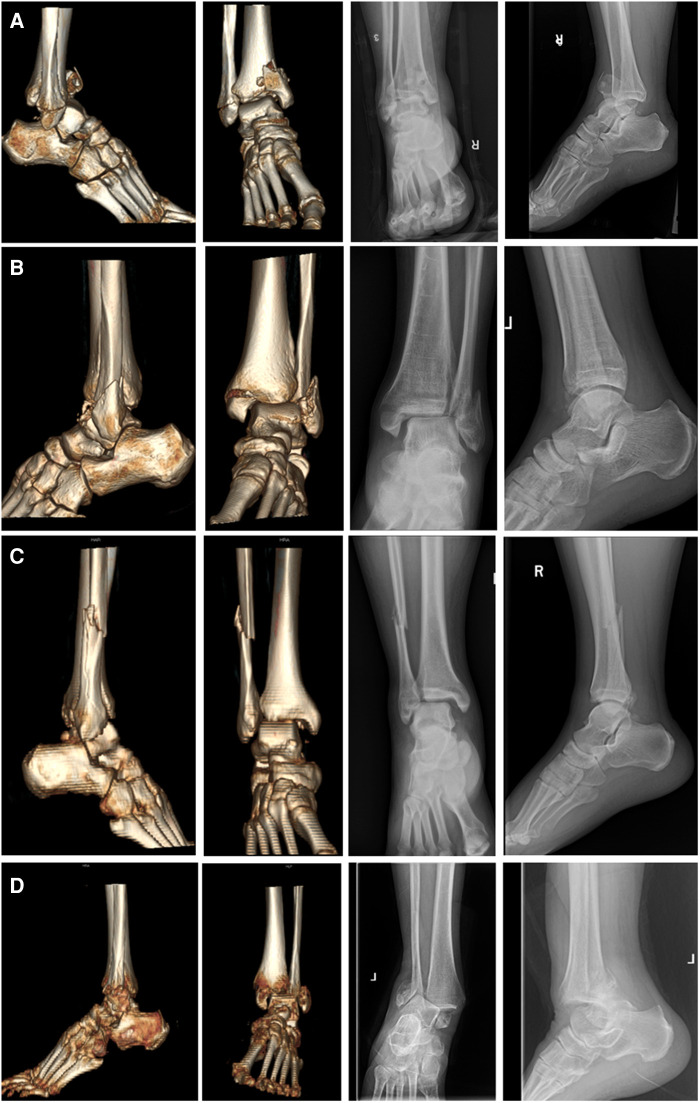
The CT reconstructions and radiographs of ankle fracture-dislocations with four different injury mechanisms according to the Lauge-Hansen's classification. (A) Supination-adduction; (B) Supination-external rotation; (C) Pronation abduction; (D) Pronation-external rotation.
